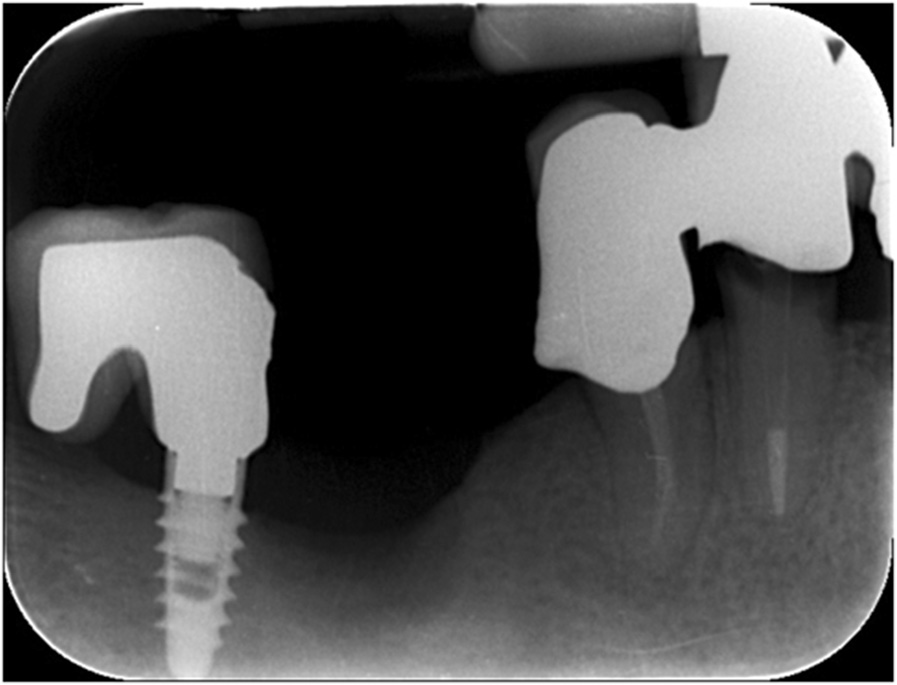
Intraoral radiography: defect after implant removal
